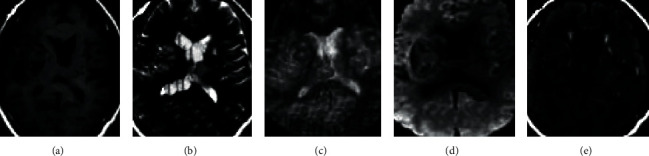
Imaging data of a patient with HICH. (a–e): T1WI, T2WI, pressurized-water T2WI, DWI, and FLAIR, respectively.
